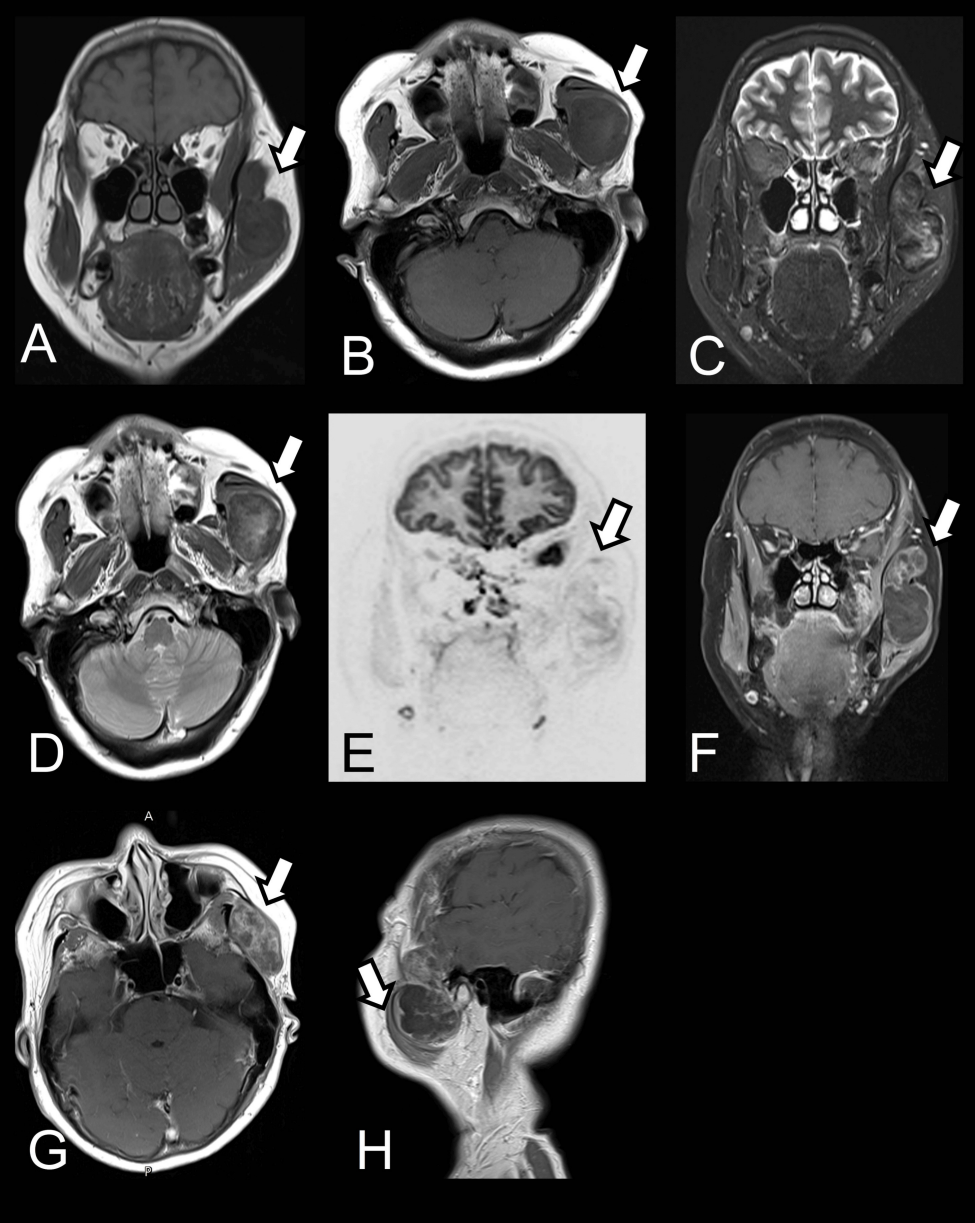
MRI. (A and B) T1-weighted images demonstrate a low signal, capsular enhanced mass around the left masticator space with the involvement of the left supratemporal fossa. (C) T2-weighted, (D) proton density, and (E) diffusion-weighted images show intralesion moderate signal without peripheral edema. (F, G, and H) Post-contrast images show heterogeneous enhancement. MRI: magnetic resonance imaging
